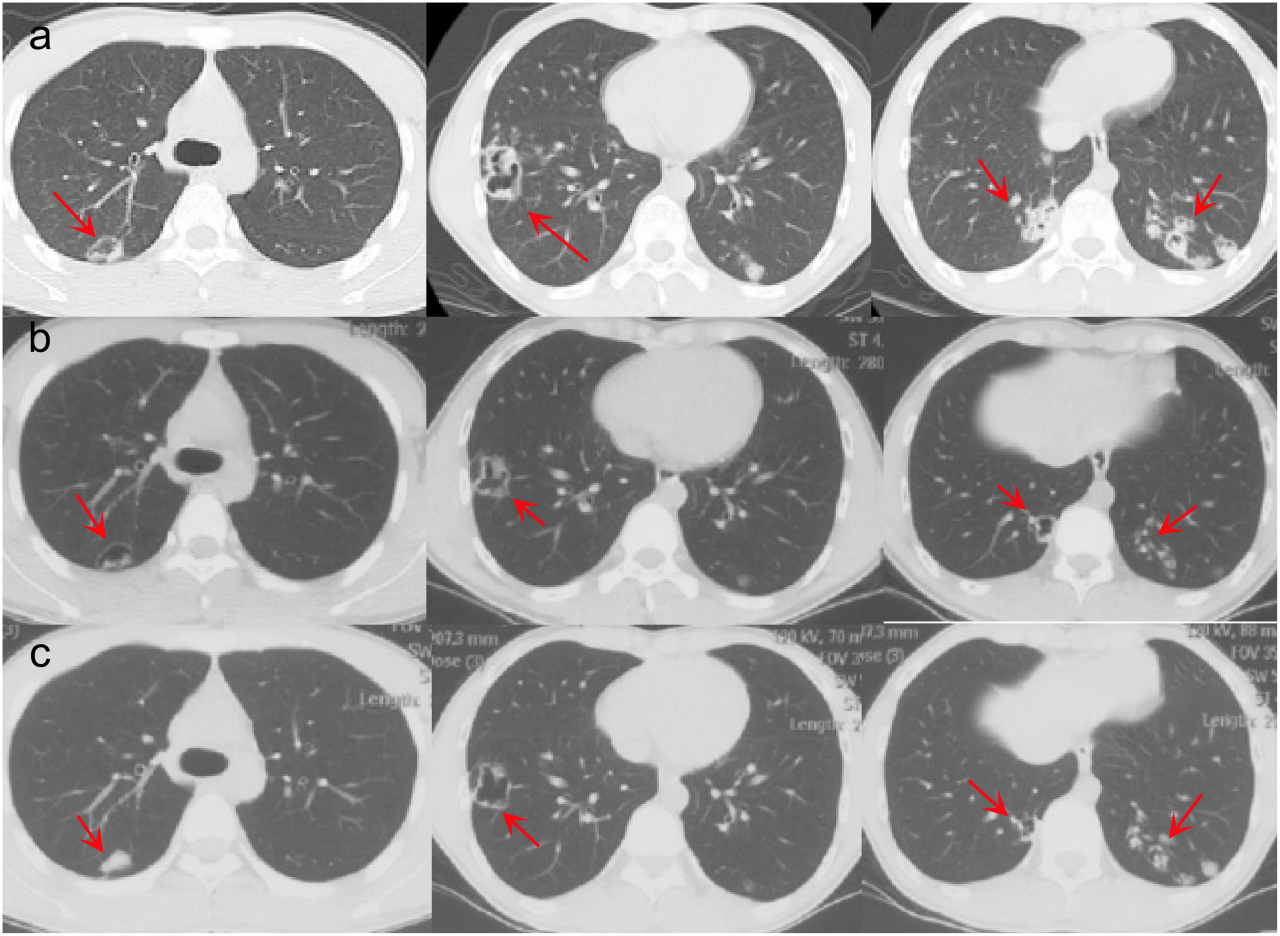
Pre- and post-therapy CT scan. (a) Chest CT before treatment. (b) Chest CT after cefotaxime sodium sulbactam treatment. (c) Chest CT after voriconazole treatment.
